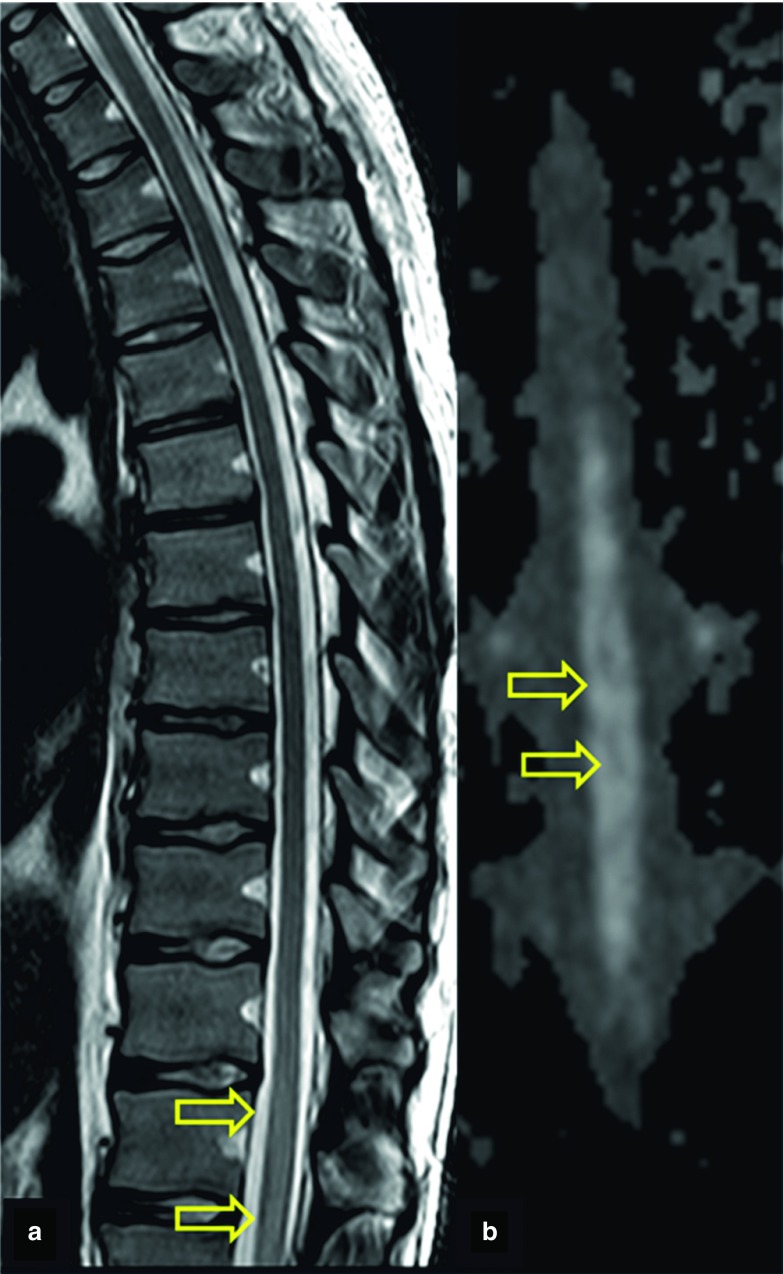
MRI 18 h following onset of symptoms. (a) The sagittal *T*_2_ weighted turbo spin-echo MR image shows oedema and swelling of the conus medullaris (arrows). (b) Low apparent diffusion coefficient is shown in keeping with restricted diffusion, which supports the diagnosis of acute ischaemia (arrows).
